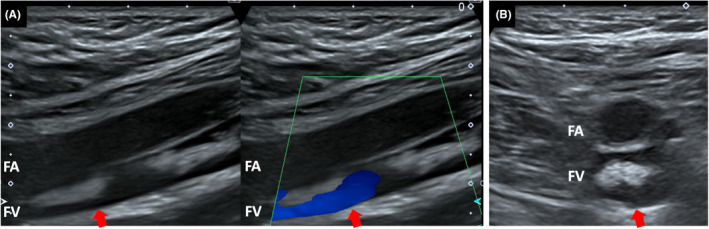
Lower extremity venous ultrasound displayed a soft tissue of 4.6 centimeters in length with high echogenicity, mobility, elasticity, and surrounding blood flow (arrow). A: Long‐axis view (left, B‐mode imaging; right, color Doppler imaging). B: Short‐axis view. FA, femoral artery; FV, femoral vein
